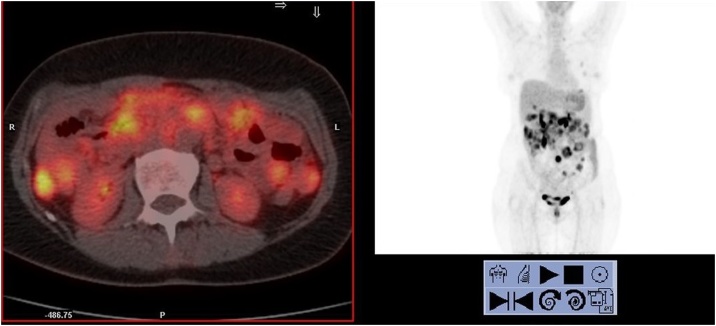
CT-PET pre adjuvant chemotherapy.
